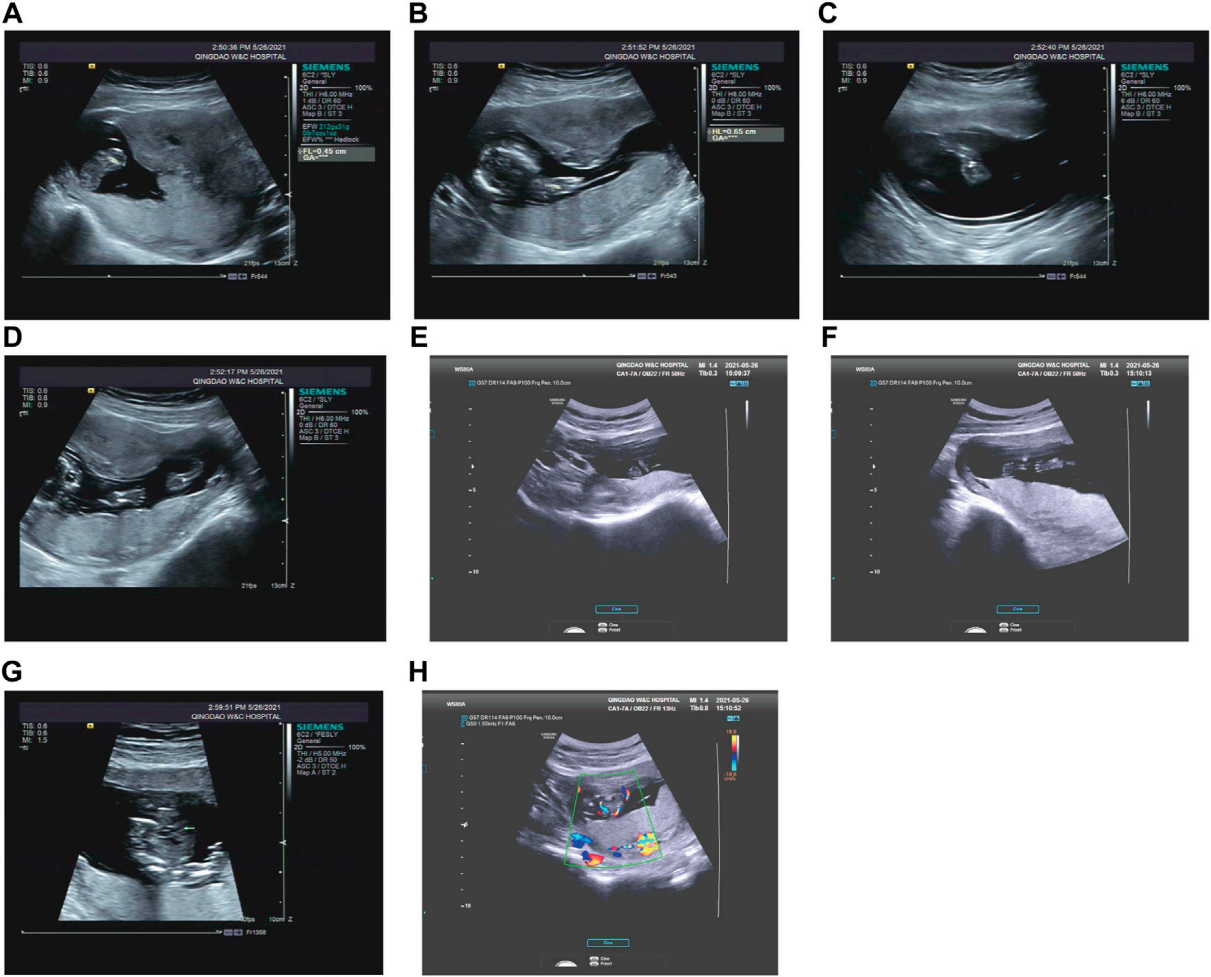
Antenatal ultrasound of foetus 3 (A) The femur length was 0.45 cm. (B) The humerus length was 0.65 cm. (C) The ulna and radius were not clearly shown. (D) The tibiofibula was not clearly visualized. (E) Polydactyly of both feet. (F) Posture abnormalities of both feet. (G) Abnormal structure of four-chamber heart. (H) single umbilical artery.
